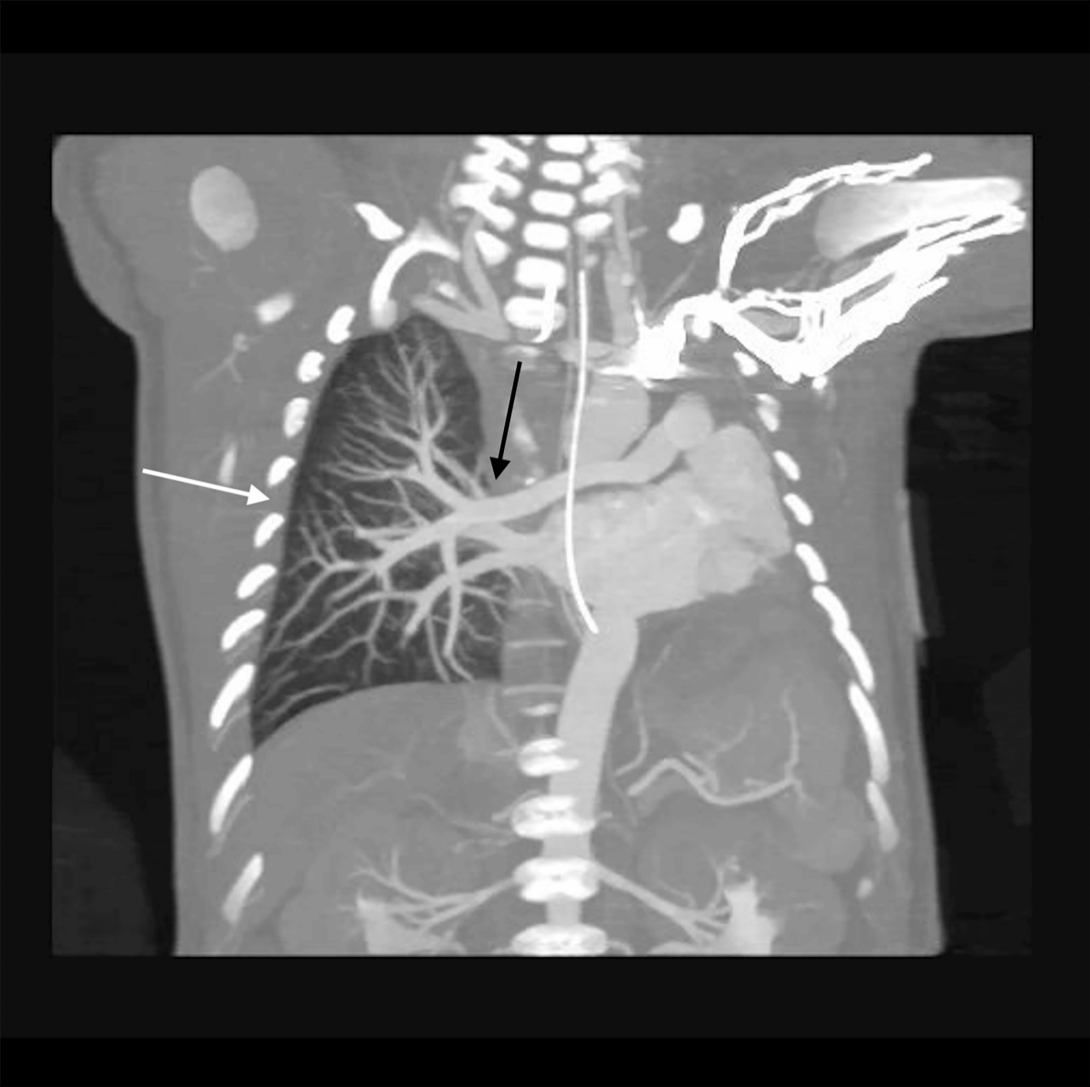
Coronal computed tomography angiography demonstrating complete left lung agenesis with absence of the left pulmonary artery and pulmonary veins The right lung (white arrow) is hyperexpanded and compensates by receiving the entire pulmonary arterial flow (black arrow).
